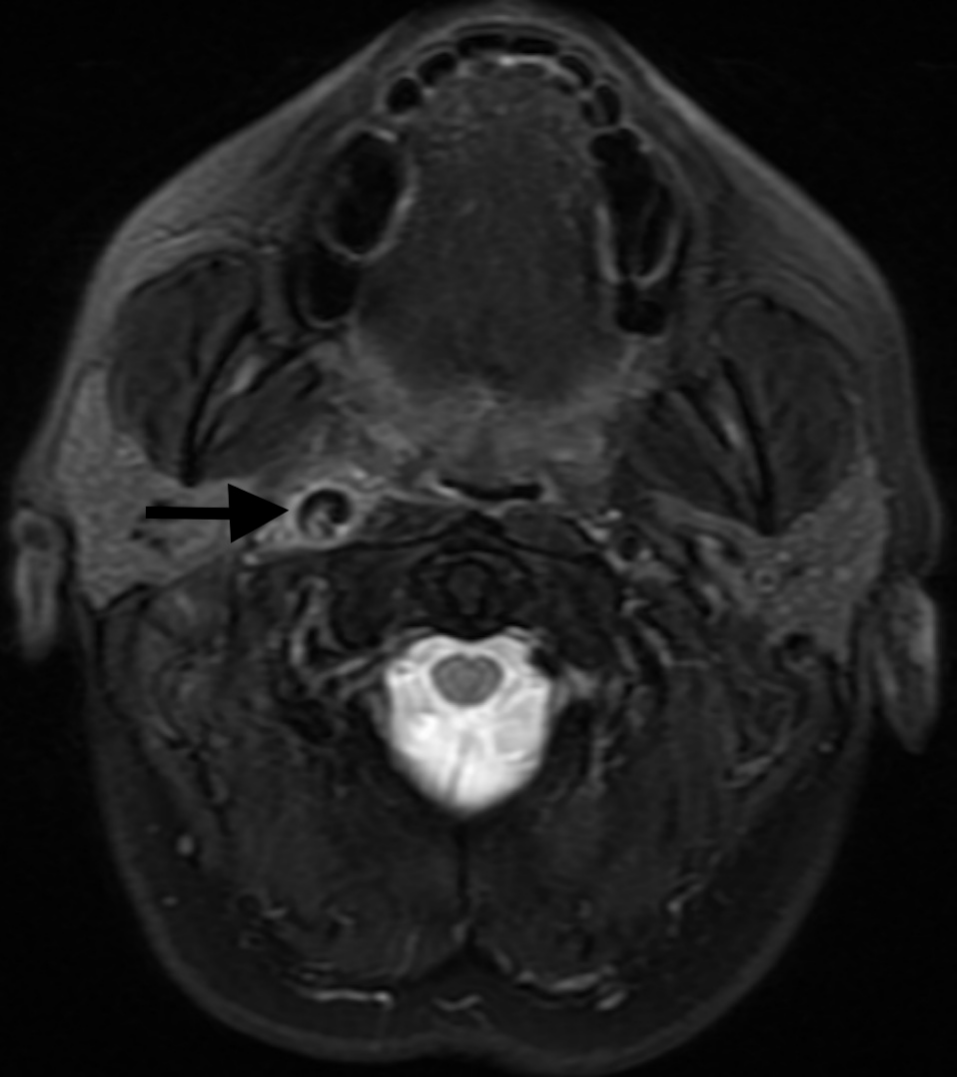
Magnetic Resonance Imaging (MRI) Brain MRI brain showing right distal internal carotid artery dissection. A notable dissection flap, along with mild inflammatory type signal changes, surrounding the carotid artery is seen (arrow).
